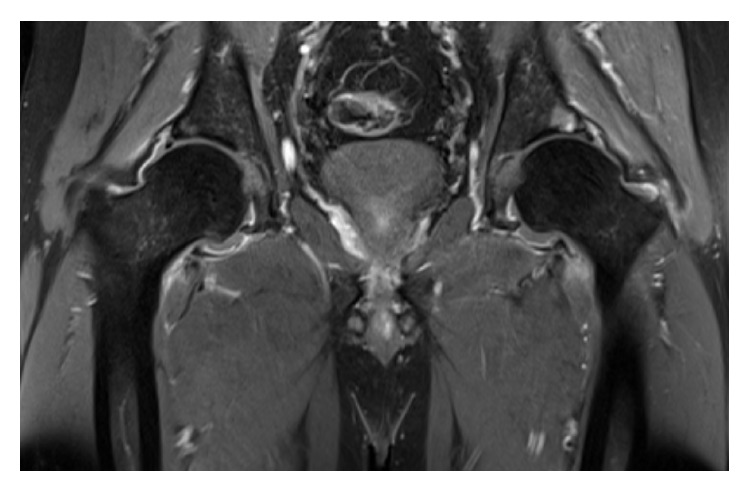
T2 weighted MRI demonstrating a hyperintense focus in the left superior acetabular rim and hip effusion and capsular edema consistent with osteomyelitis and septic arthritis.
